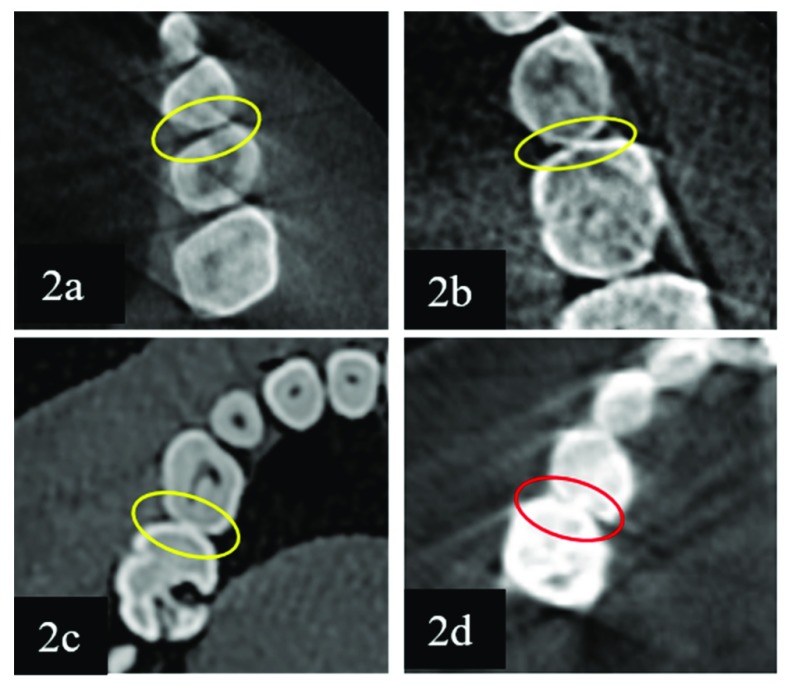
CBCT images showing different types of contact areas between primary molars. (
